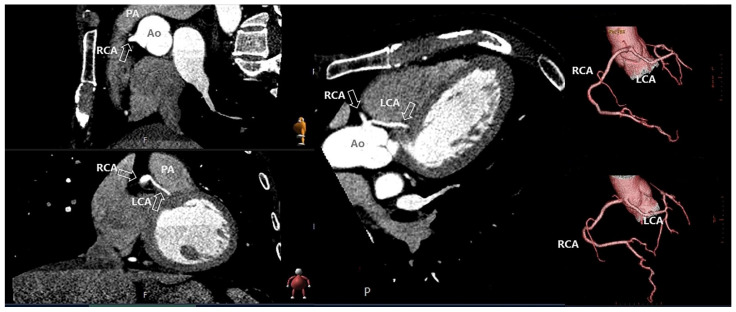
Coronary anomaly visible and diagnosed with computed tomography. The figure shows the anomalous origin of the left coronary artery (LCA) from the right sinus of Valsalva with the LCA between the aorta and the pulmonary artery. Ao: aorta; PA; pulmonary artery; RCA; right coronary artery.
